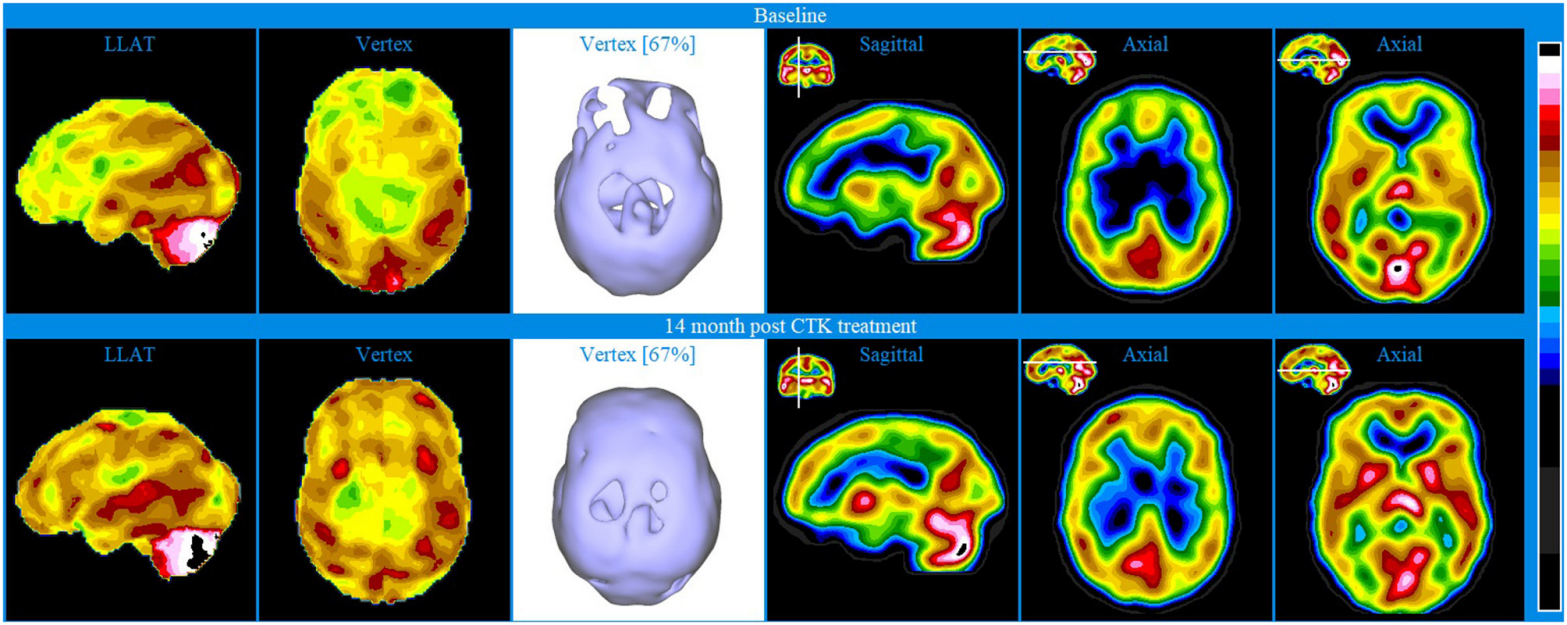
Brain SPECT images for Patient IV. Top line relates to brain SPECT images at baseline. Bottom line shows SPECT images 14 months following 30 sessions of CTK treatment. In each row (from left to right) two stereotactic surface projections of the left lateral (LLAT) and vertex, one iso-surface image of the vertex, one sagittal slice and two axial slices.
